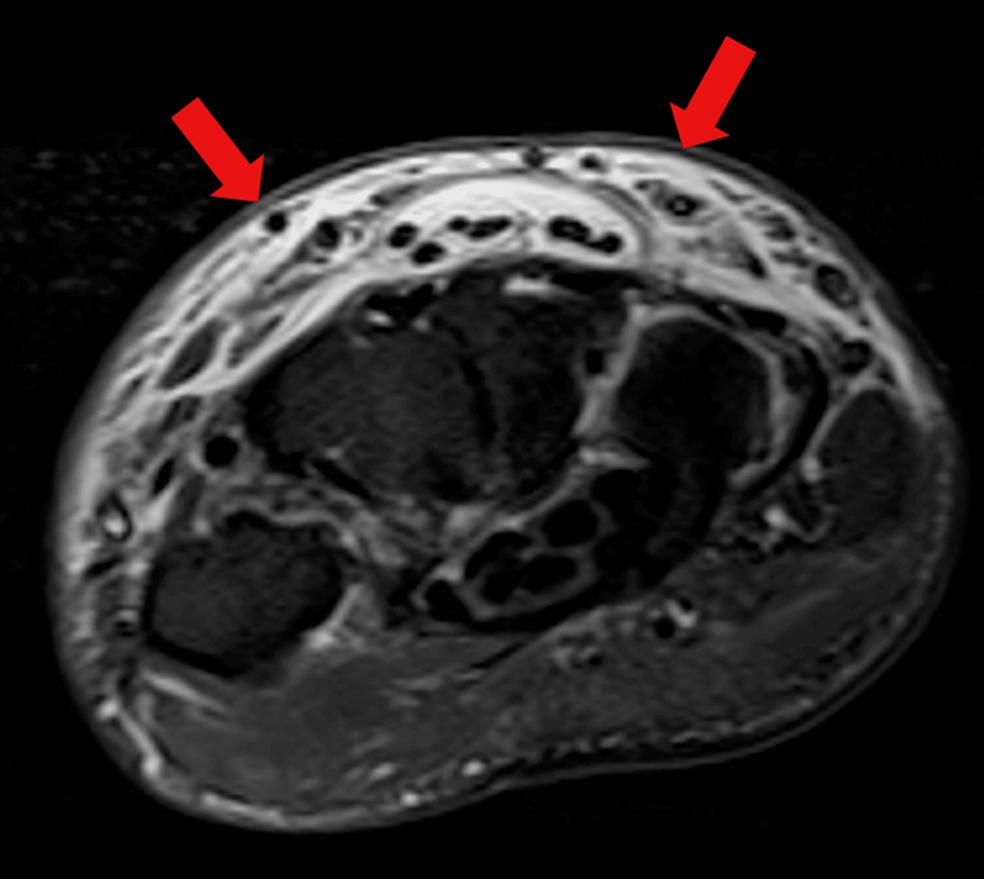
MRI axial STIR image showing dorsal edema STIR: Short Tau Inversion Recovery
